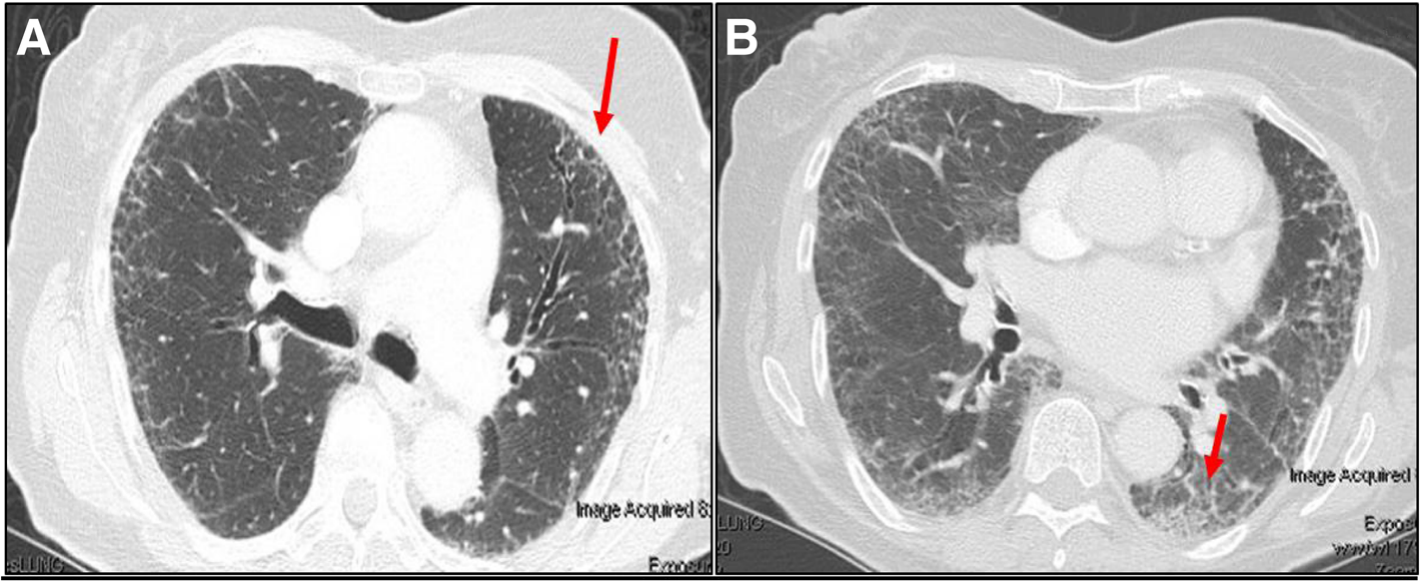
a Computed tomography demonstrates honeycombing (red arrow) distributed in the left lower lobe. Bronchial thickening is observed in the left lung. b Chest CT revealing subpleural ground glass opacities (red arrow)
